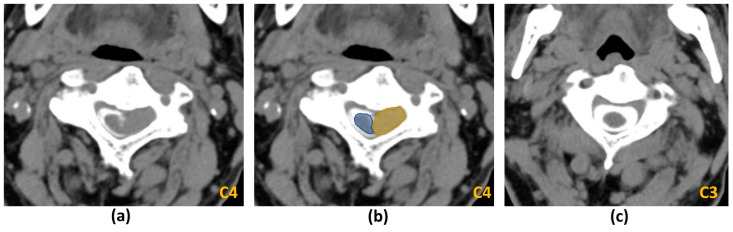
CT Myelogram of a lung cancer patient with suspected metastatic spinal cord compression (MSCC) at the level of C4, without annotations (a) and with annotations (b). The epidural disease is indicated by the yellow colourwash and the spinal cord by the blue colourwash in (b). At this level, the epidural tumour abuts the spinal cord with partial obliteration of the contrast-opacified subarachnoid space (Bilsky 2 MSCC). More superiorly at the level of C3 (c), no evidence of MSCC or epidural disease is seen.
